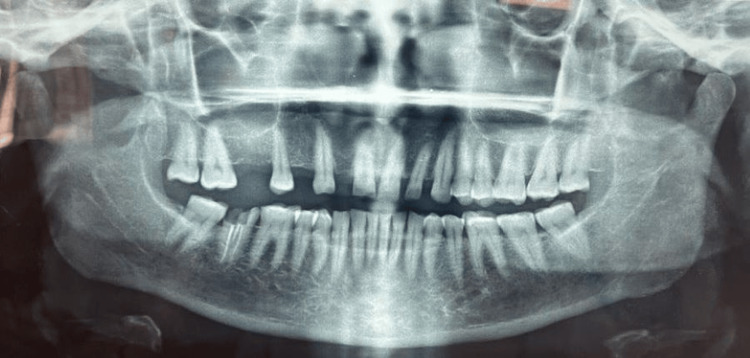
Orthopantomogram Image Credit: Prasanna Sonar
